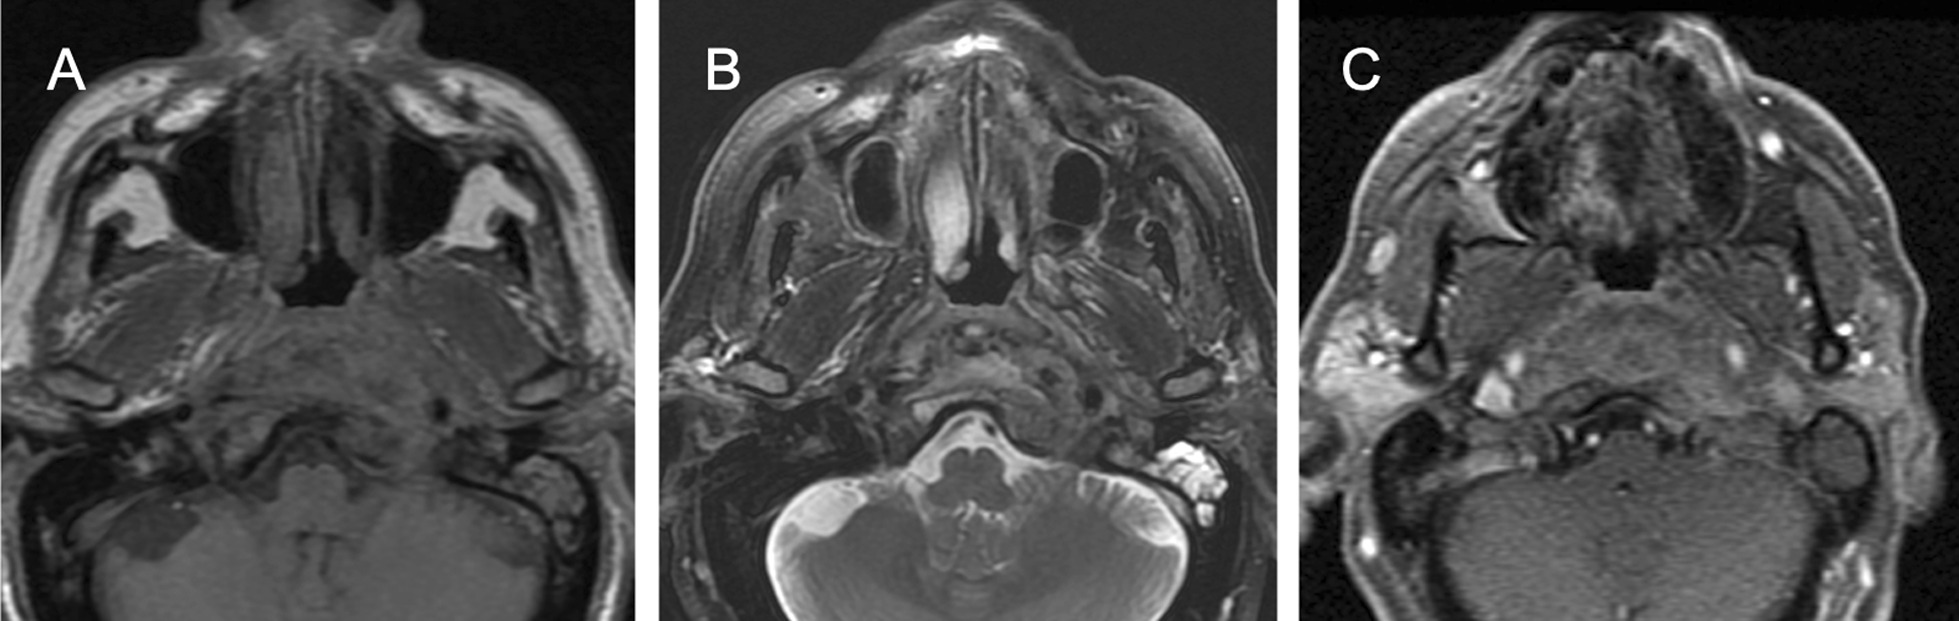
MR images obtained one week later confirmed the presence of an infiltrative hypointense on T1-weighted (a) and T2-weighted (b) images mass with irregular enhancement on post-contrast images (D). Infiltration of adjacent bone marrow is also observed
